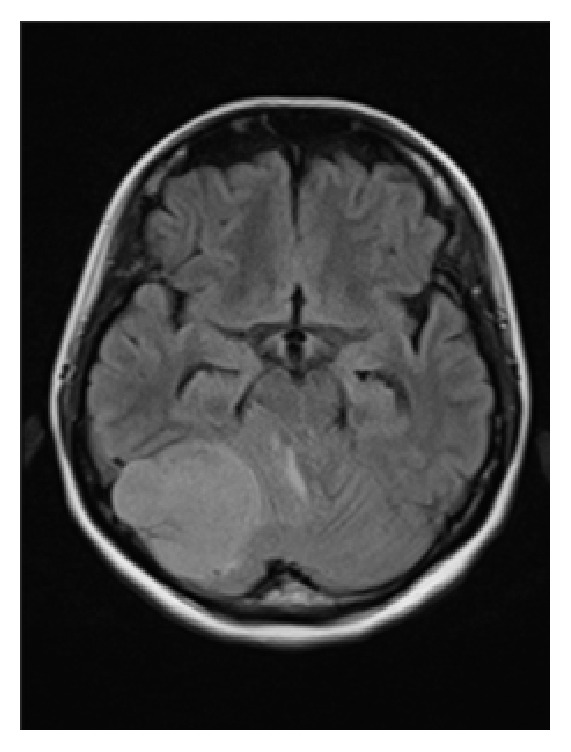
FLAIR MRI of the brain. Compression by the mass toward the brainstem caused deformation.
